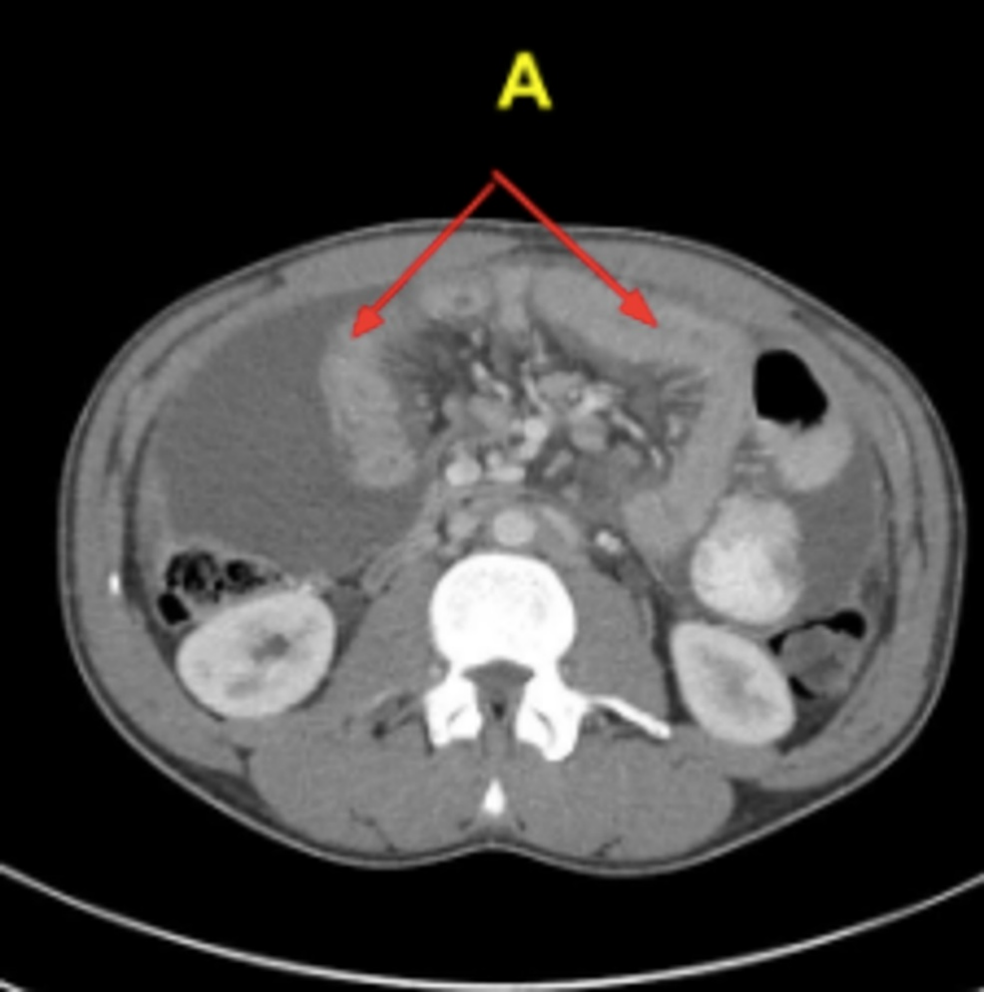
Axial CT image showing mural thickening and diffuse edema of the small bowel, most notably the jejunum (marked by red arrows, labeled A).
